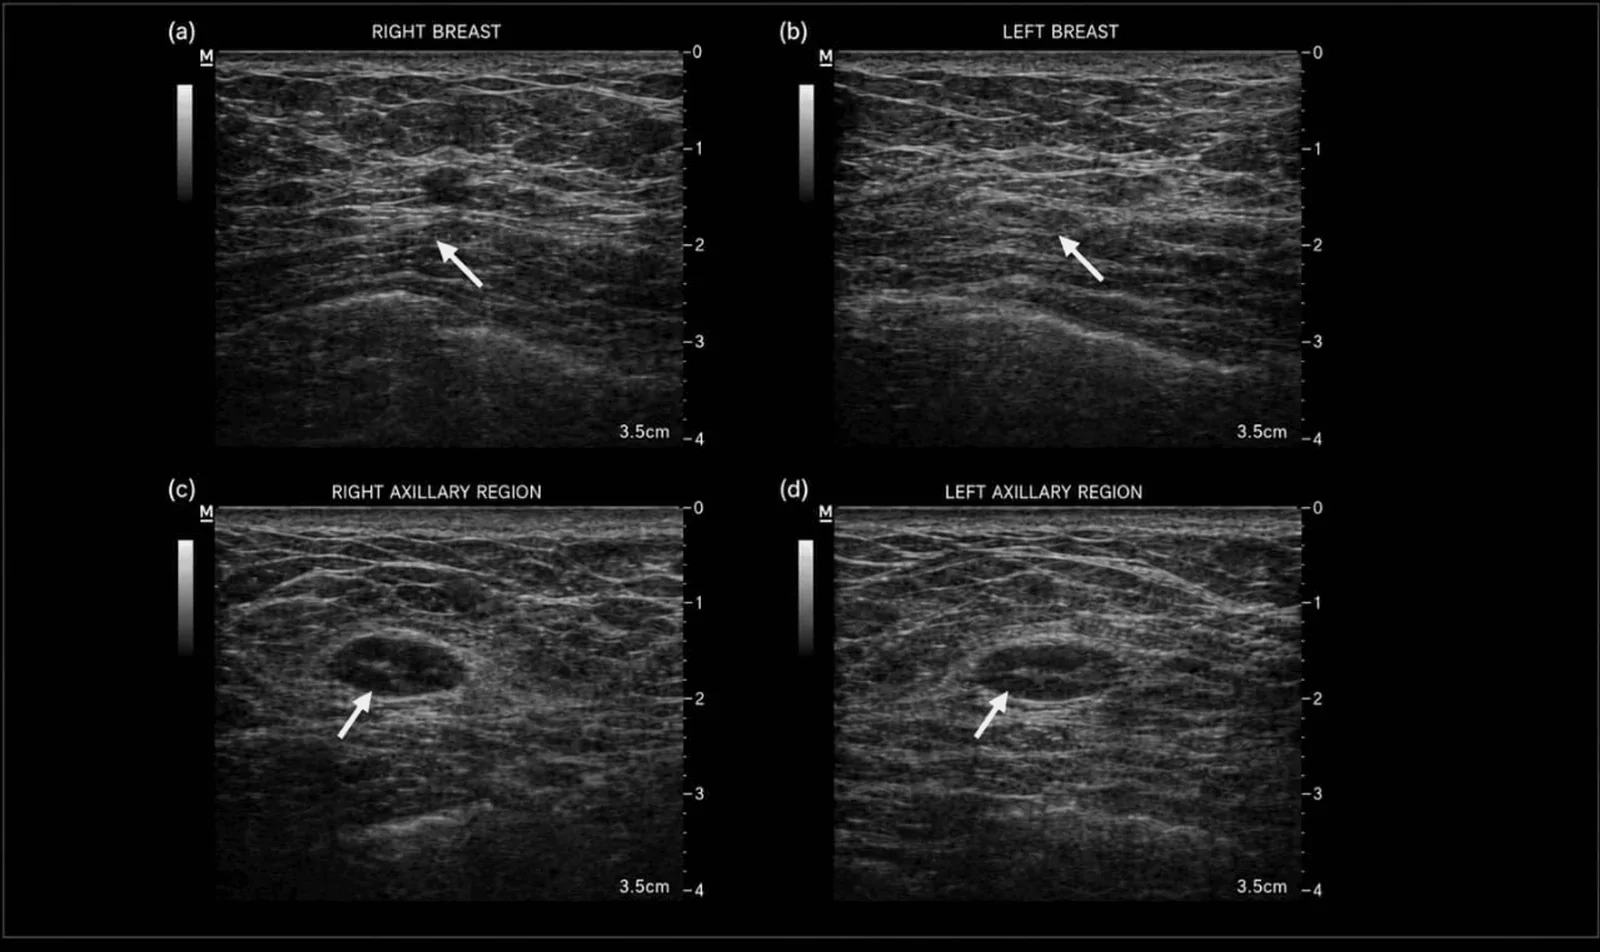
Ultrasonographic appearance of bilateral gynecomastia showing subareolar fibroglandular proliferation. (a) Right breast ultrasound showing diffuse fibroglandular parenchymal tissue involving the subareolar region with maximum thickness measuring 1.3 cm (arrow). (b) Left breast ultrasound showing diffuse fibroglandular parenchymal tissue involving the subareolar region with maximum thickness measuring 1.25 cm (arrow). (c) Right axillary ultrasound showing a subcentimetric lymph node (arrow). (d) Left axillary ultrasound showing a subcentimetric lymph node (arrow).
